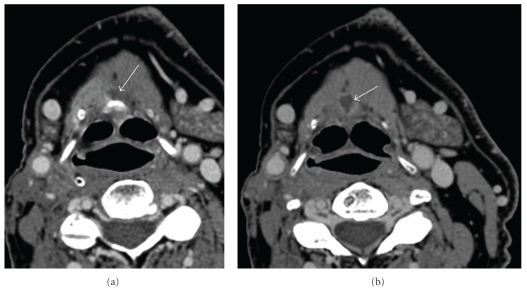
Enlarging thyroglossal duct cyst: (a, b) Axial contrast-enhanced CT at the level of the hyoid bone (soft tissue window) demonstrates a thyroglossal duct cyst (arrow) which has enlarged following radiation therapy and is containing more fluid centrally (arrow).
